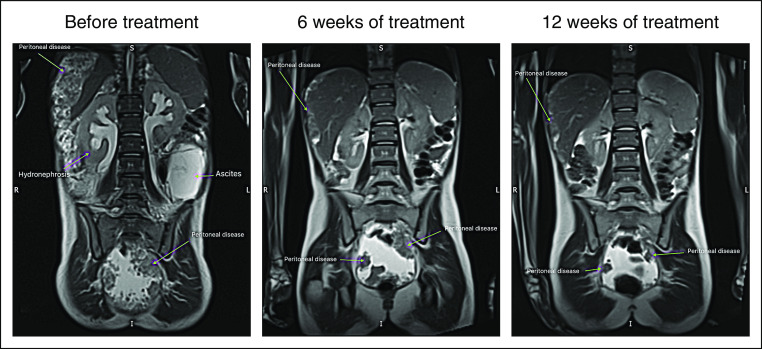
Gadolinium-enhanced magnetic resonance imaging scans of the abdomen before and at 6 weeks and 12 weeks after starting ceritinib. Images before treatment demonstrate large-volume ascites, bilateral hydronephrosis, and widespread peritoneal soft tissue deposits particularly within the pelvis (all findings marked by green arrows). Follow-up imaging 1 month after treatment was started showed a significant reduction in tumor bulk in these areas and reduction in volume of ascites. Hydronephrosis has resolved. At 12 weeks after treatment, there is a further reduction in the volume of peritoneal disease and ascites.
